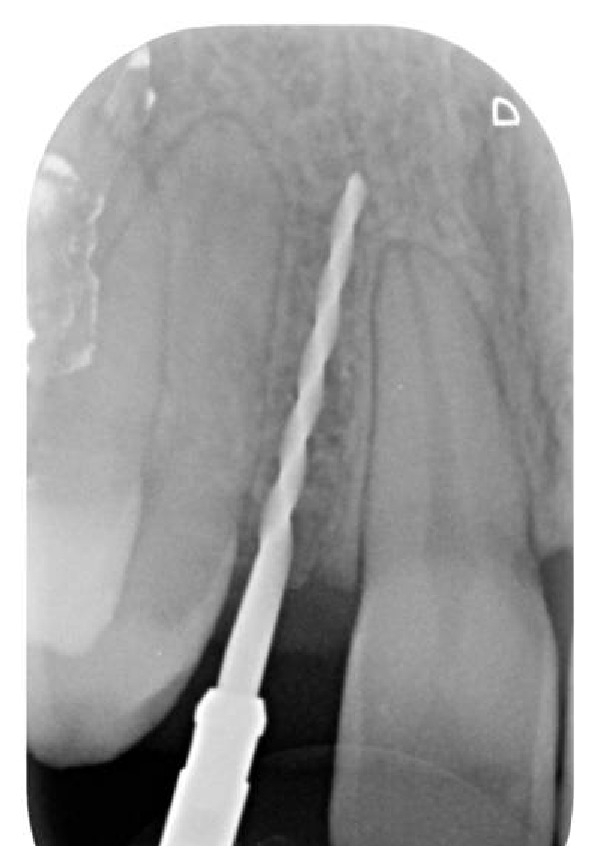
Radiographic examination of the correct inclination in site to the left.
